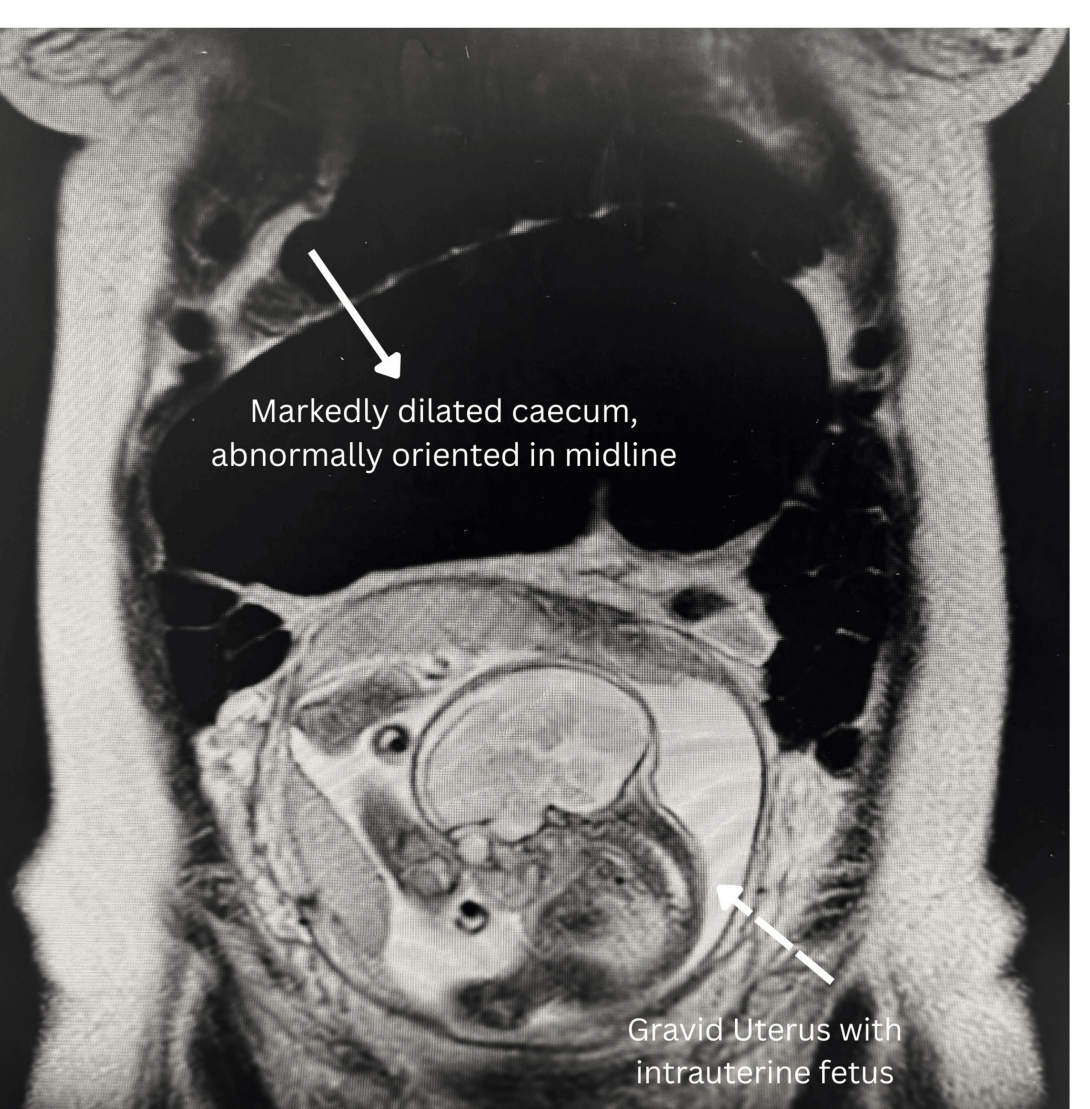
T2-weighted coronal magnetic resonance imaging (MRI) of the abdomen demonstrating a markedly dilated, abnormally oriented cecum positioned in the midline, consistent with caecal volvulus( solid white arrowhead). The gravid uterus with an intrauterine foetus is visualised inferiorly(broken arrowhead). MRI: magnetic resonance imaging
